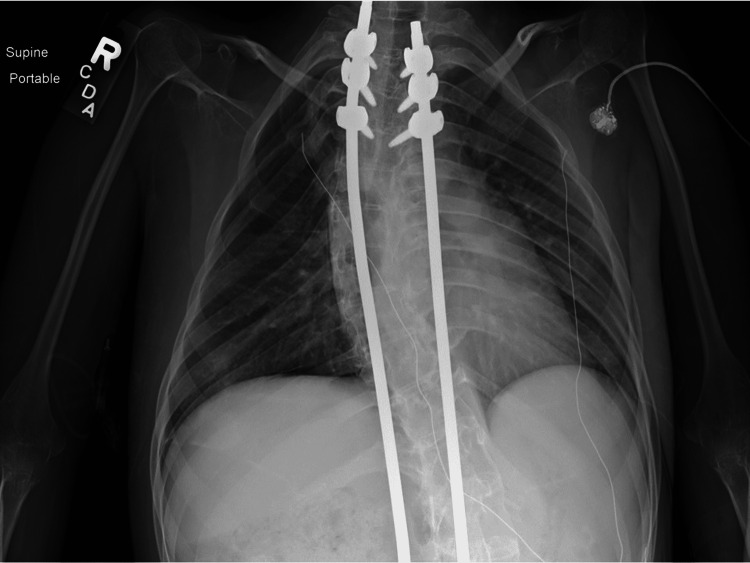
Last chest radiograph performed, showing almost complete resolution of the second pneumothorax after second blood patch.
